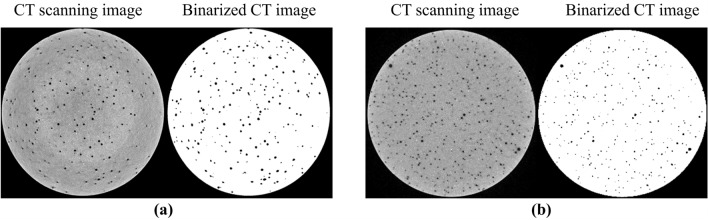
Binarized CT images of the porous structure. (a) Net slurry cement; (b) Liquid silicon cement.
